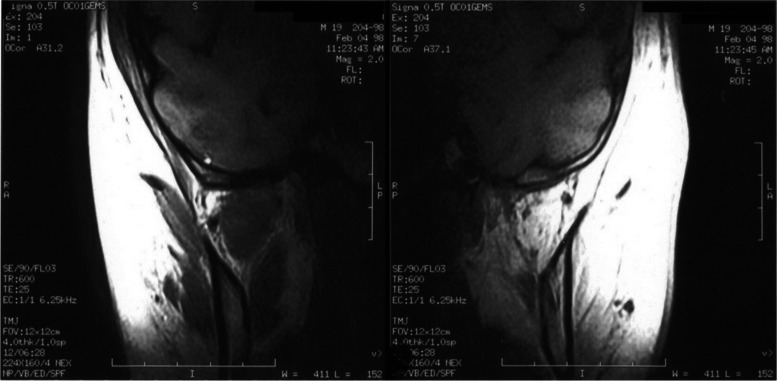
MRI coronal T1-weighted scans. a Right side: the masticatory muscles appear normal. b Left side: masticatory muscles appear severely hypotrophic
